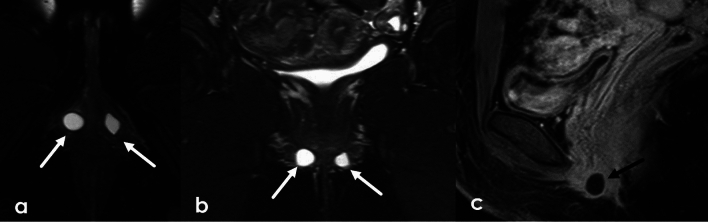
34-Year-old female undergoing MRI for uterine fibroids with incidentally noted Bartholin gland cysts. Axial and coronal fat saturated T2WI demonstrates bilateral cystic lesions located posterior and lateral to the vaginal introitus (white arrows). Sagittal contrast enhanced T1WI demonstrates nonenhancement of a cyst (black arrow). Note location below the pubic symphysis
